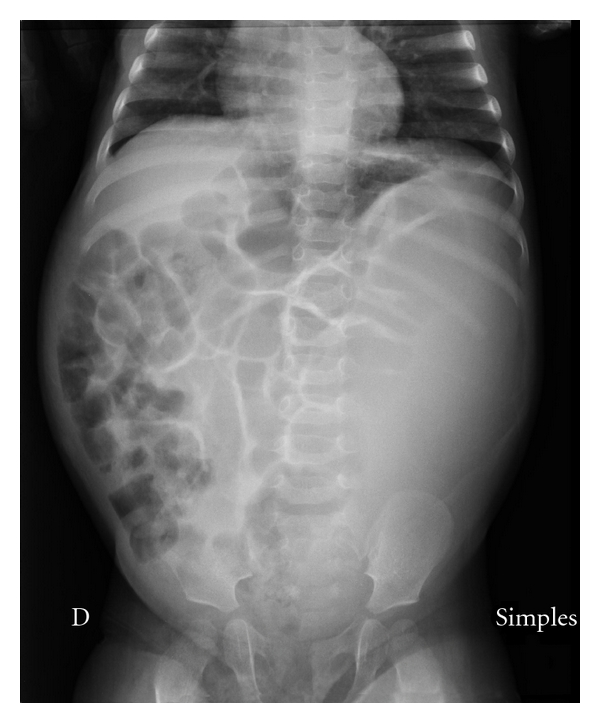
Abdominal plain radiography showing elevation of both hemidiaphragms, prominent gaseous distension of bowel loops, which are completely deviated to the right side of the abdomen, and homogeneous opacity of the left hemiabdomen.
